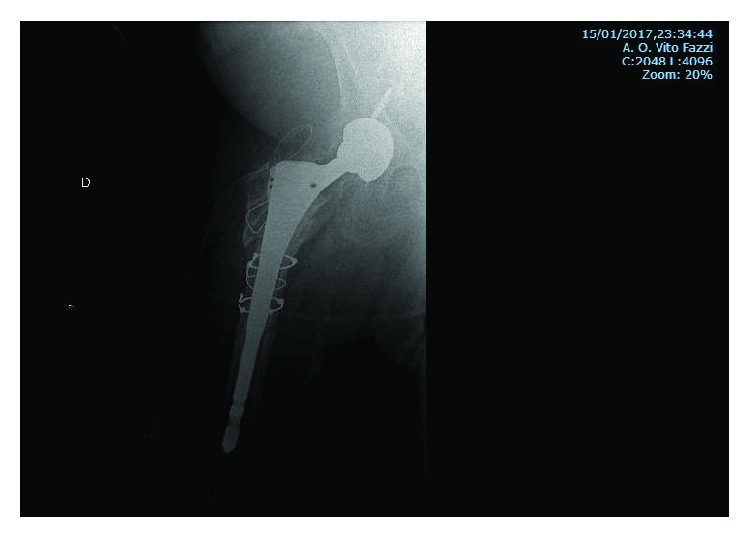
Postsurgery X-ray, after nail removal and replacement with revision THA with two screws to fix the cup and three metal cable cerclages to prevent the breakage during the implant of the stem.
